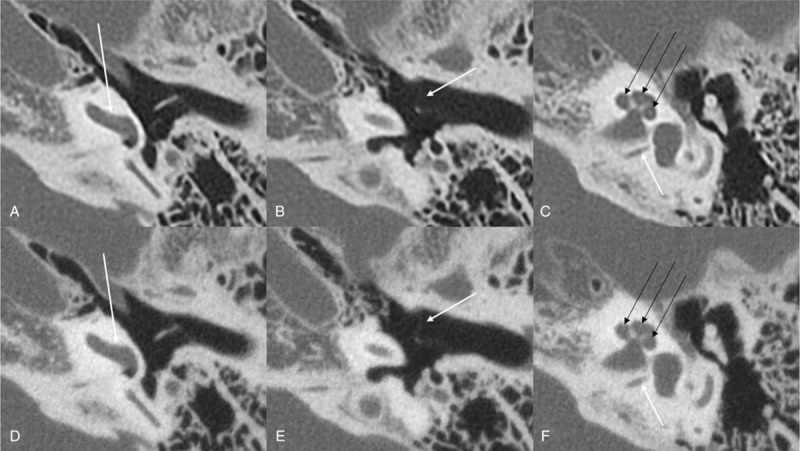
Temporal bone CT in a patient reconstructed with hybrid iterative reconstruction (HIR) (AIDR3D enhanced mild mode; A–C) and with model-based iterative reconstruction (MBIR) (Forward projected model-based Iterative Reconstruction SoluTion (FIRST) BONE mild mode; D–F). The spiral osseous lamina is clearly delineated in HIR (A; score 3), whereas it is nearly invisible in MBIR (D; score 1). The tympanic membrane is visible but blurred, both in HIR (B; score 2) and in MBIR (E; score 2). The singular canal is well-delineated both in HIR (white arrow in C; score 3) and in MBIR (F; score 3). Note that the spiral osseous lamina is also well-delineated in HIR (C; black arrows) and invisible in MBIR (F; black arrows). Two radiologists graded all 3 structures in an identical manner in this case.
